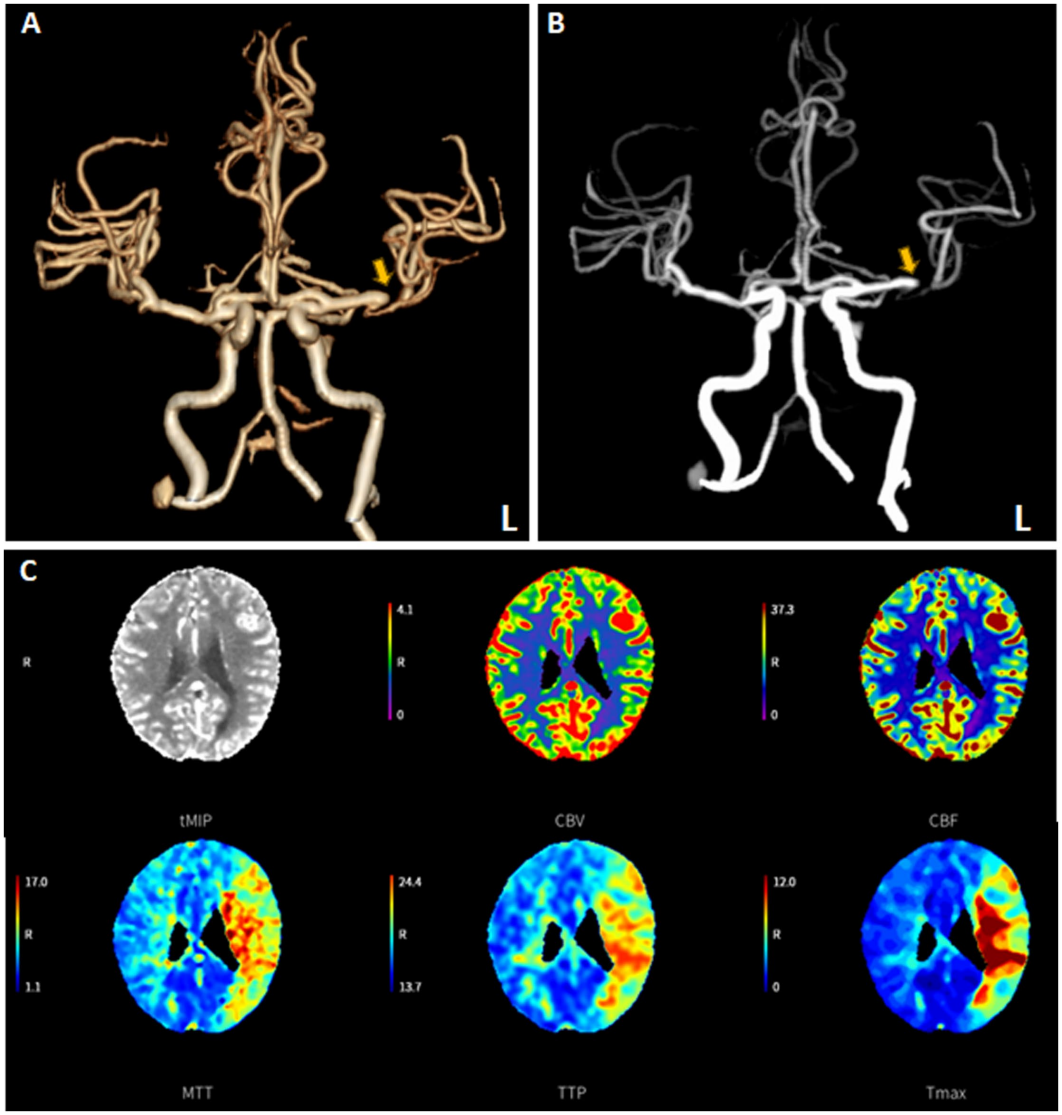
The “one-stop-shop” computed tomography (CT) images of an emergent admission patient presenting with a National Institutes of Health Stroke Scale of 21. A flat-detector CT scan was performed to exclude intracranial hemorrhage. CT angiography (CTA) demonstrated the distal M1 occlusion of the left middle cerebral artery (A,B). CT perfusion (CTP) imaging revealed the presence of perfusion mismatch at the corresponding cerebral tissue (C).
